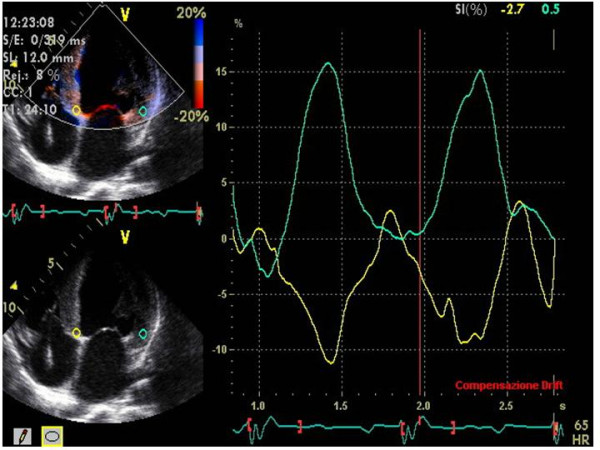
Strain (%) of basal posterior septum and lateral wall in apical 4-chamber view. Lateral wall shows an abnormal relaxation (positive sign of its curve) during systole, with a motion that is opposite to that of the basal posterior septum.
